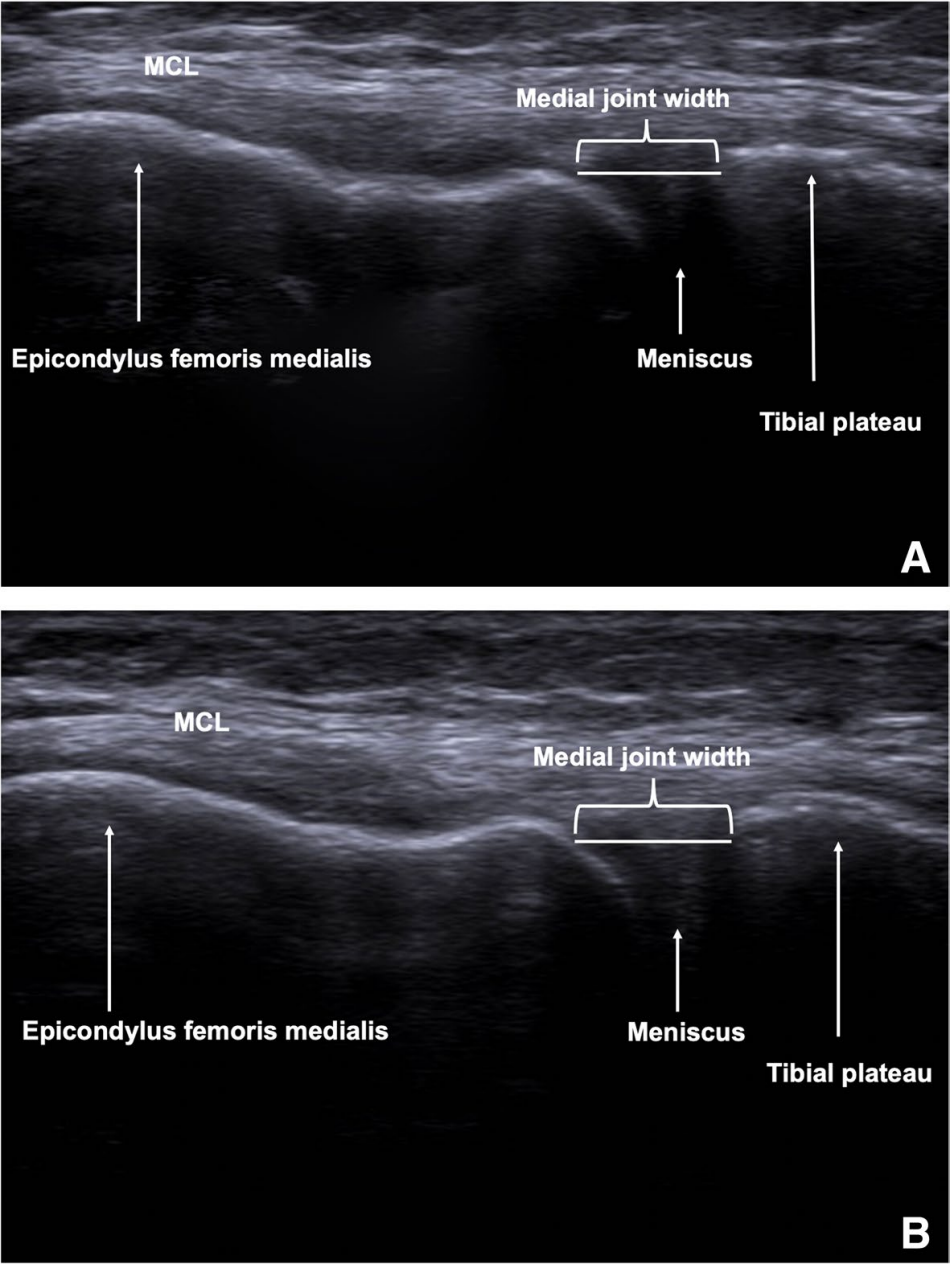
Ultrasound image of the medial joint space width in 30° knee flexion in unloaded condition (1A) and in standardized loaded condition (15 daN) with TELOS fixation device (1B). White arrows show medial femoral epicondyle, medial meniscus, and tibial plateau. A white line is positioned between femoral and tibial margins where medial joint width can be measured in millimeters. MCL, medial collateral ligament
